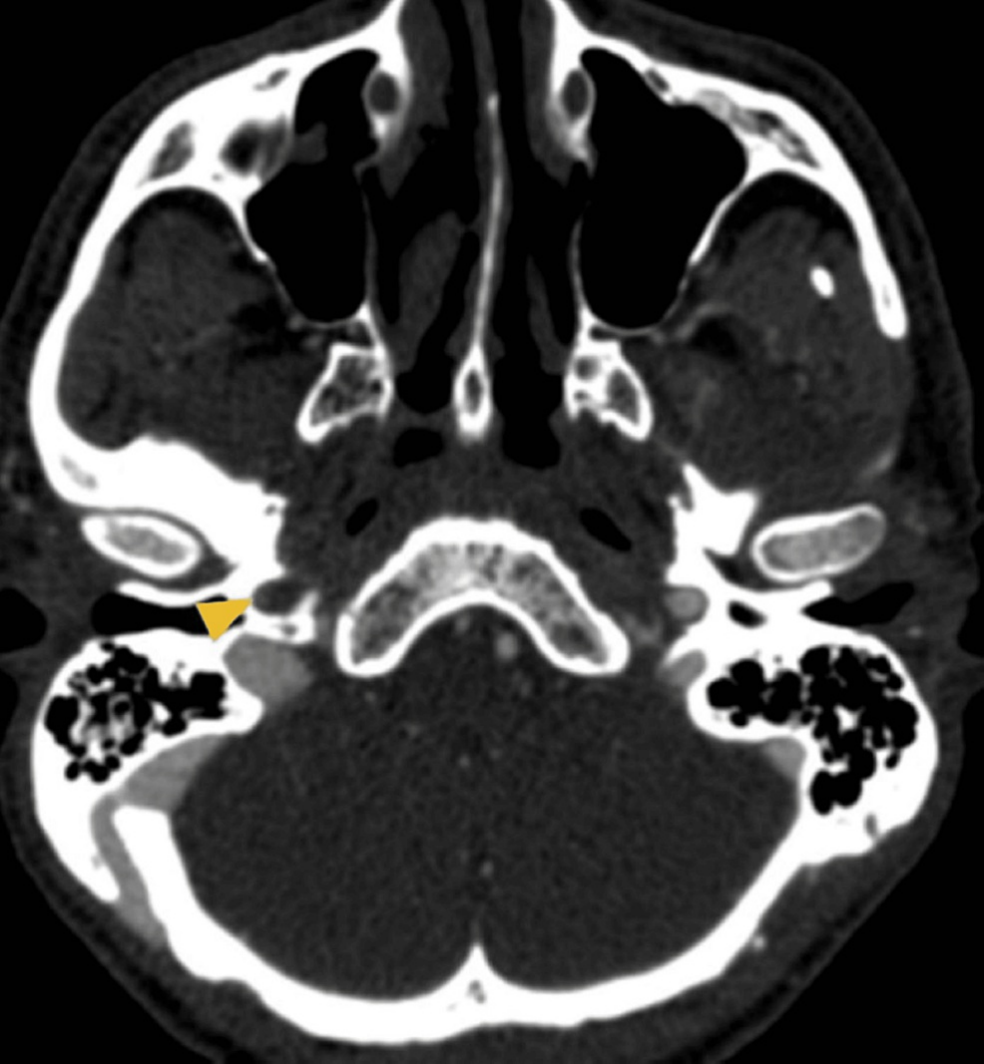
Axial cerebral CT angiography showing occlusion in cervical-petrous transition of the right internal carotid artery due to dissection
